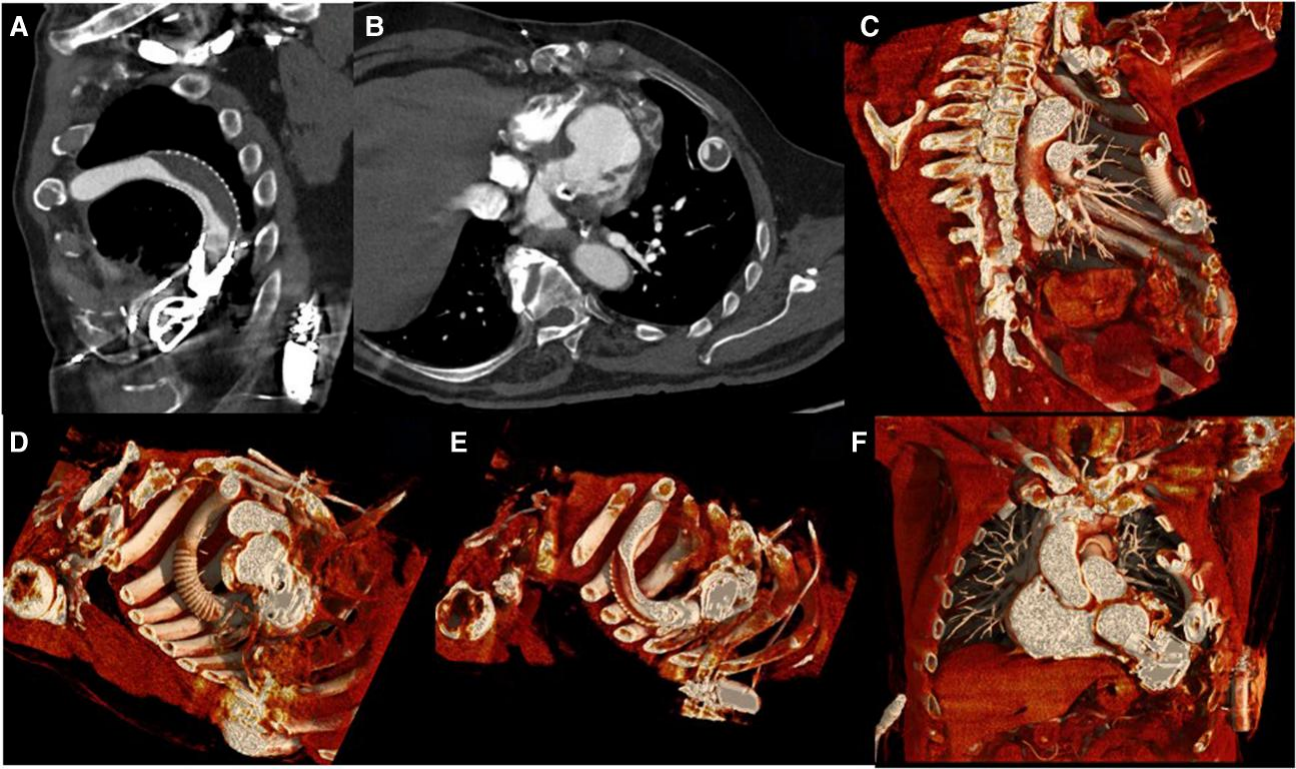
Computed tomography angiography and cinematic rendering of LVAD outflow tract compression from biodebris accumulation. (*A*) Two-dimensional oblique sagittal reformatted CTA image centred at the point of maximal compression in the LVAD outflow tract. The outflow cannula courses through the thorax with biodebris build-up (white arrow) causing external compression of the inner cannula. (*B*) Two-dimensional double orthogonal luminal view of the LVAD outflow graft in the region of biodebris accumulation. Crescent-shaped biodebris causes narrowing of the mid-portion of the inner cannula lumen (white arrow). (*C*) Three-dimensional cinematic rendering of the LVAD outflow cannula demonstrating the general course from the pump housing to the ascending aorta. The area of luminal compression in the mid-outflow graft is indicated (white arrow). The outflow graft anastomosis to the ascending aorta is marked (white arrowhead). Separate colour settings differentiate the biodebris, inner cannula lining, and blood pool. (*D*) Three-dimensional cinematic rendering with segmentation demonstrating the external features of the outflow cannula. The extent of biodebris accumulation along the external surface of the outflow graft is indicated (white arrow). The inflow cannula at the left ventricular apex is marked (arrowhead). (*E*) Cut-surface segmentation with the outflow cannula partially cut-open to demonstrate biodebris collection with associated extrinsic compression of the inner lumen (white arrow). This view clearly demonstrates that the obstruction results from external compression rather than intraluminal thrombus, as the biodebris is located between the outer and inner cannula walls. The invaginated appearance of the inner lining under pressure from biodebris build-up is evident. (*F*) Cinematic rendering assessment of the LVAD inflow cannula demonstrating device position and orientation. The inflow cannula tip is positioned within the left ventricular apex (white arrow) with the cannula oriented toward the interventricular septum, representing somewhat suboptimal positioning in the setting of myocardial thinning from prior infarcts. A portion of the outflow cannula is also visualized.
